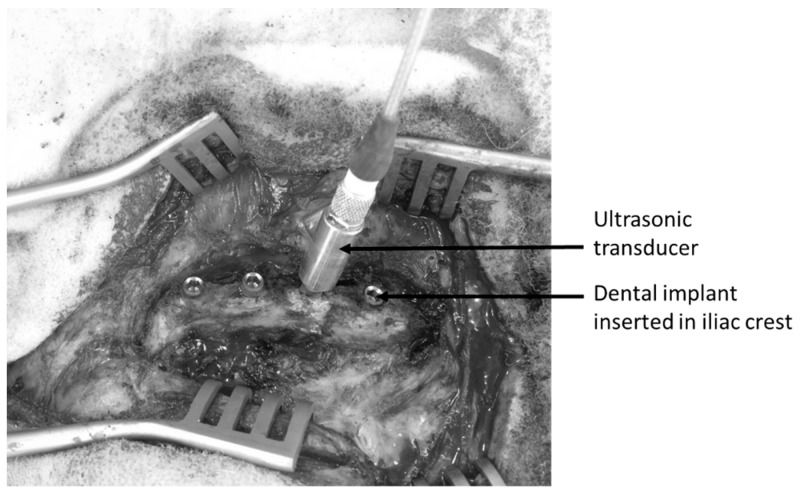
Measurement configuration of the ultrasonic indicator using the ultrasonic transducer screwed into a dental implant.
